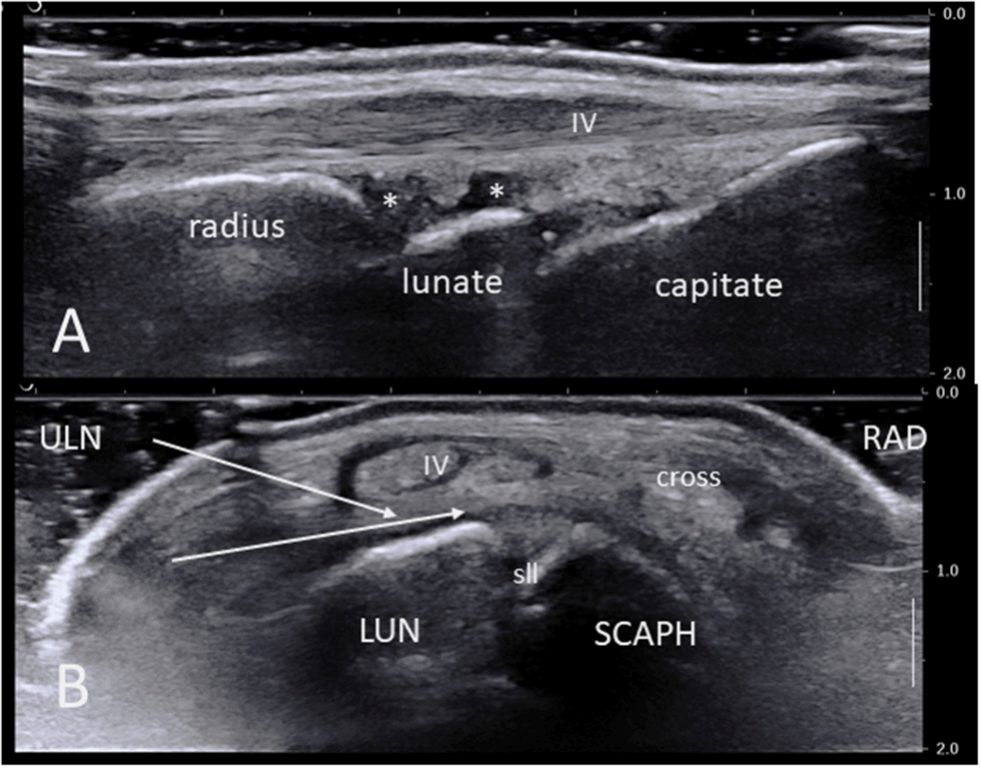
(A) Ultrasound long-axis scan of a normal wrist joint using a high frequency linear transducer. Note the radius, lunate, and capitate bone surfaces, the synovial recesses (asterisks), the IV extensor tendon compartment and its retinaculum. (B) Short axis ultrasound scan of a normal right wrist. The area of maximal synovial thickening usually lies posterior and radially to the scapho-lunate junction. White arrows show the two most common needle paths between extensor tendons compartments V and IV and VI and V. IV: fourth extensor tendon compartment; cross: crossing point between compartments III and II; LUN, semi-lunate bone; RAD, radial side; SCAPH, scaphoid bone; sll, scapho-lunate ligament; ULN, ulnar side.
